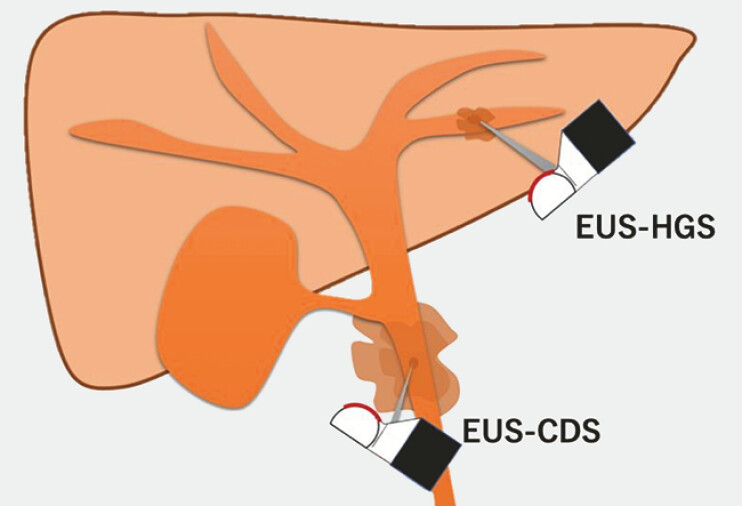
The liver parenchyma surrounding the bile duct prevents bile leakage in endoscopic ultrasound guided-hepaticogastrostomy (EUS-HGS), whereas endoscopic ultrasound guided-choledochoduodenostomy (EUS-CDS) has a higher risk of bile leakage because of the absence of surrounding organ protection.
